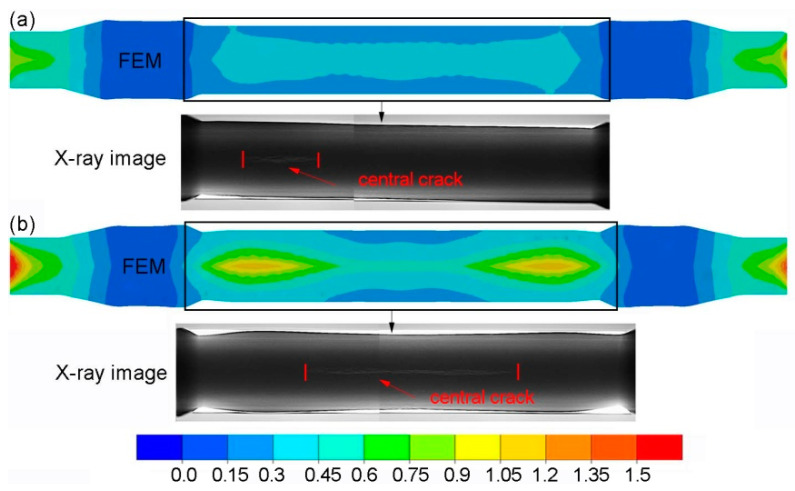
Distribution of the Cockcroft–Latham damage criterion and X-ray image of the rolled axle: (a) with traditional tools; (b) with multi-wedge tools.
